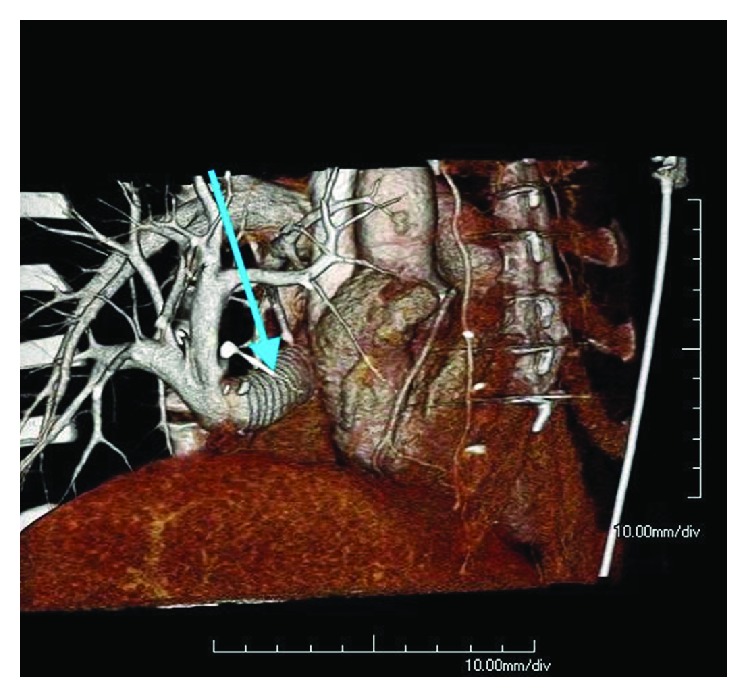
3D volume rendering of postoperative CT angiogram from a right anterior oblique view. Arrow pointing to the artificial baffle, draining the scimitar vein, aiming posteriorly toward the left atrium.
